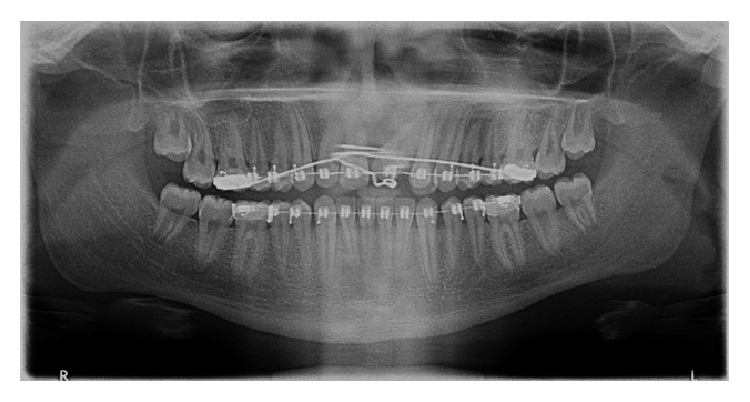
Follow-up panoramic radiograph depicting erupted maxillary central incisor.
